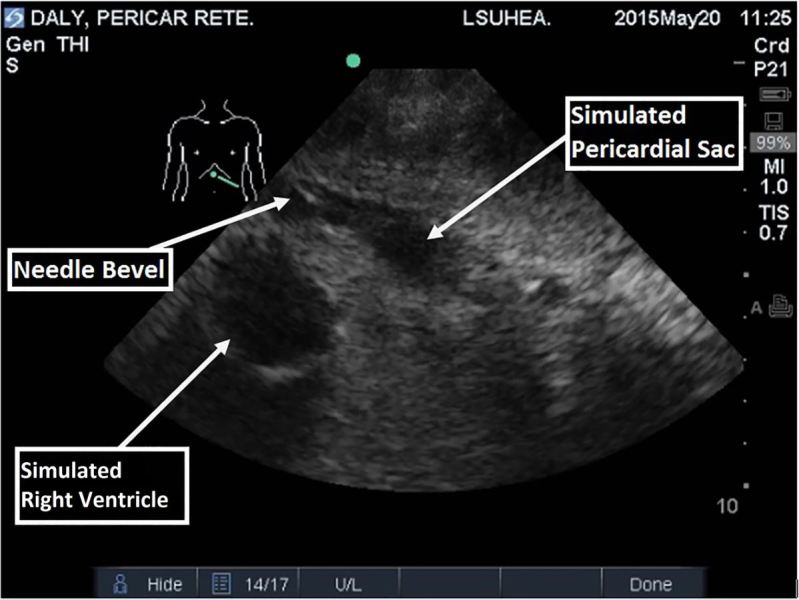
Residents performed ultrasound-guided pericardiocentesis using a model constructed of inexpensive materials.
